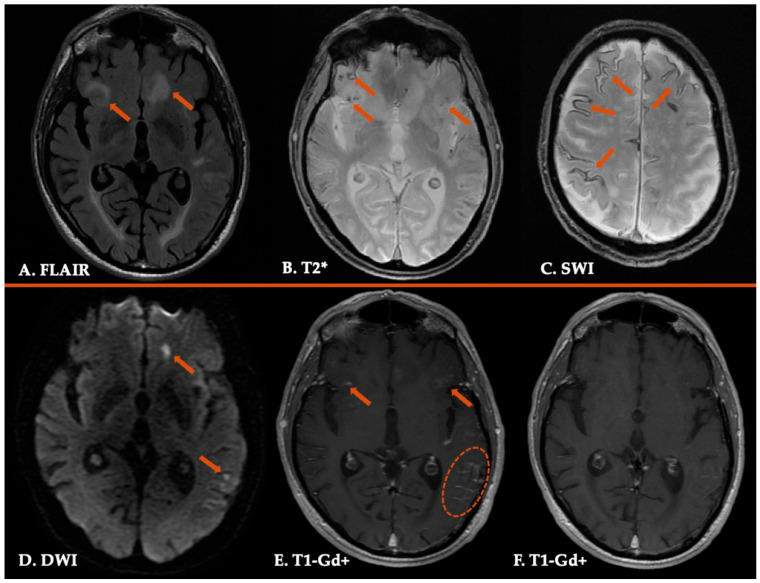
Neuroimaging findings in a patient with Cerebral Amyloid Angiopathy-related inflammation. Three-dimensional fluid-attenuated inversion recovery sequence (FLAIR) revealing multiple bilateral asymmetric white matter hyperintensities (Panel (A)). Axial T2* images showing multiple lobar cerebral microbleeds (Panel (B)) and disseminated cortical superficial siderosis (Panel (C)). Diffusion-weighted imaging (DWI) sequence (Panel (D)) demonstrating multiple cortical and subcortical ischemic lesions (Panel (D)). T1-weighted imaging showing extensive leptomeningeal gadolinium enhancement (Panel (E)) with complete resolution following intravenous treatment with methylprednisolone (Panel (F)).
